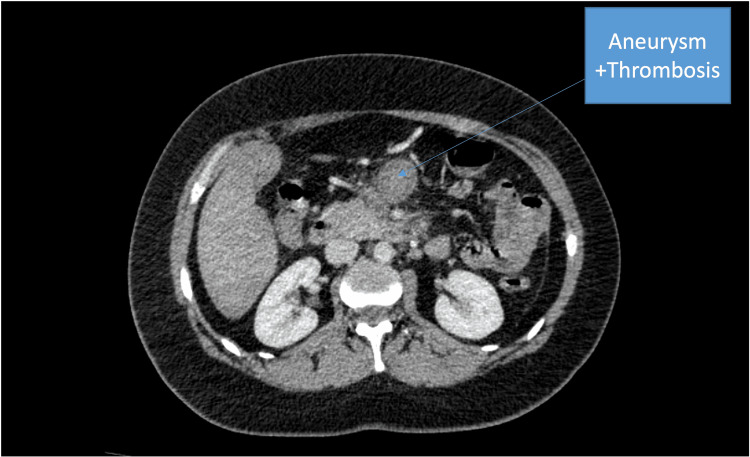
CT angiography showing a saccular, thrombotic aneurysm involving the superior mesenteric vein (SMV).
